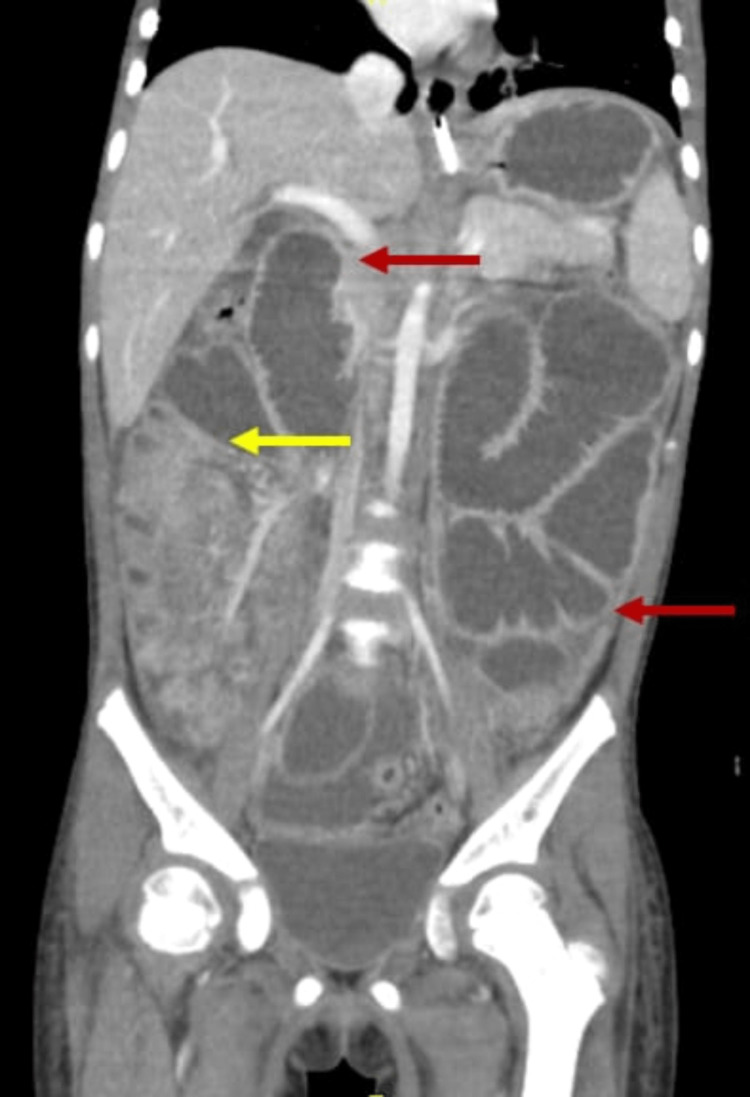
Coronal plane of repeated CT scan at second Emergency Department presentation showing a diffuse dilatation of proximal bowel (red arrows), and collapsed distal bowel. Distal bowel obstruction with transitional zone in the distal jejunum/proximal ileum (yellow arrow).
